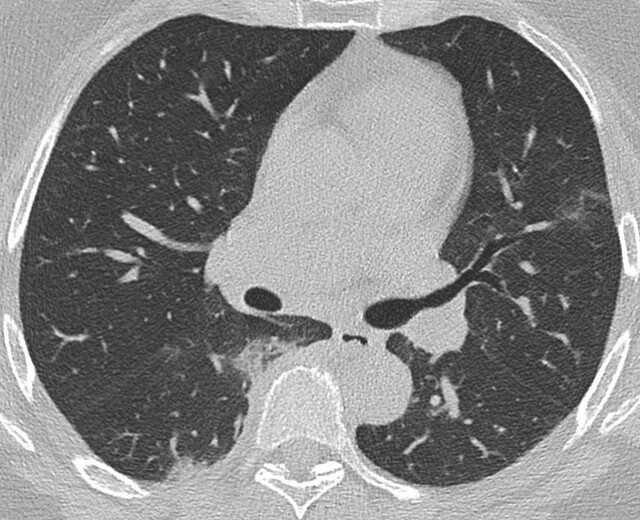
CT of a 38-year-old female with fever, cough, dyspnoea and anosmia at presentation: subpleural/peripheral GGOs. GGO, ground-glass opacity.
